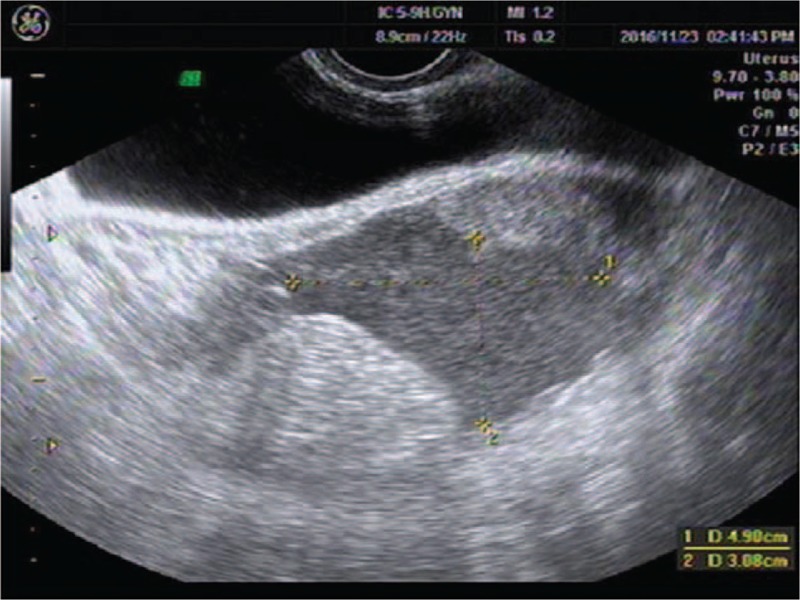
Ultrasonographic evidence of hemoperitoneum was present with a large amount of free fluid within the pouch of Douglas.
